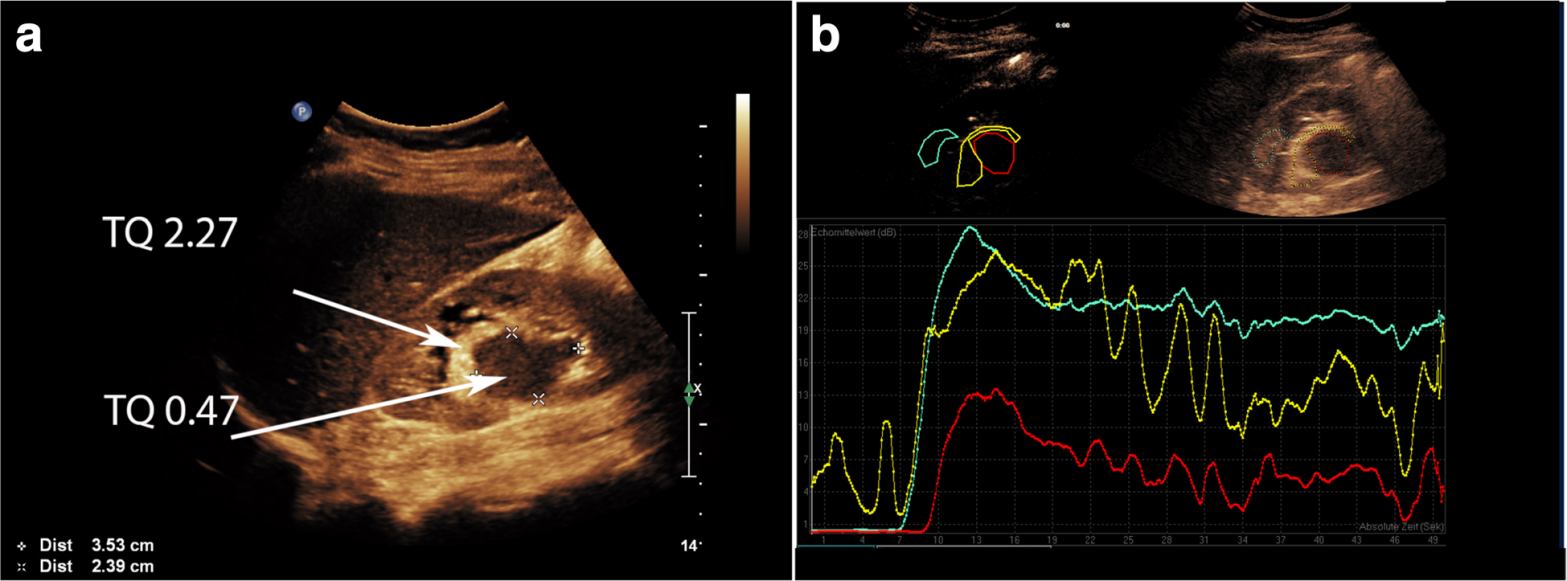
a B-mode ultrasound reveals two areas with different echogenicity within the tumor: Outer rim is hyperechoic with an echogenicity tumor to normal kidney cortex ratio (TQ) of 2.27 (a value larger than 2.0 is characteristic for angiomyolipoma) and an inner hypoechoic portion (TQ 0.47) [11]. b Time intensity curves (TIC) of the contrast enhanced ultrasound (CEUS) showing an early and strong enhancement of the hyperechoic outer rim (yellow line), similar to renal cortex (blue line), and a later and weaker enhancement of hypoechoic inner portion (red line). The strong enhancement of the outer tumor rim is clearly seen on the ventral side
